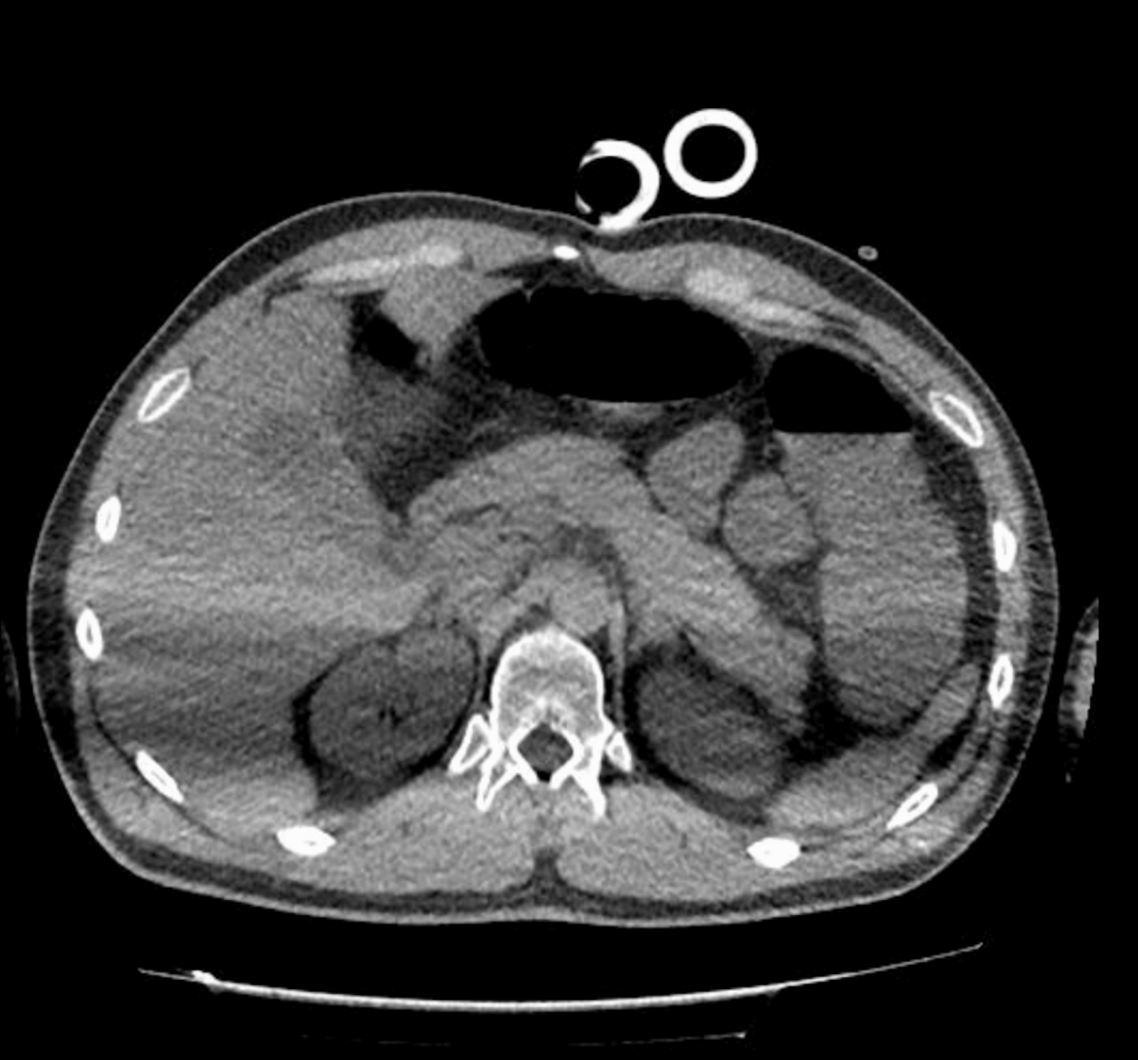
Abdominal CT scan showing no abnormalities. CT scan of the upper abdomen and pelvis was performed using a multidetector helical technique. Images were obtained before and after intravenous administration of the contrast medium.
